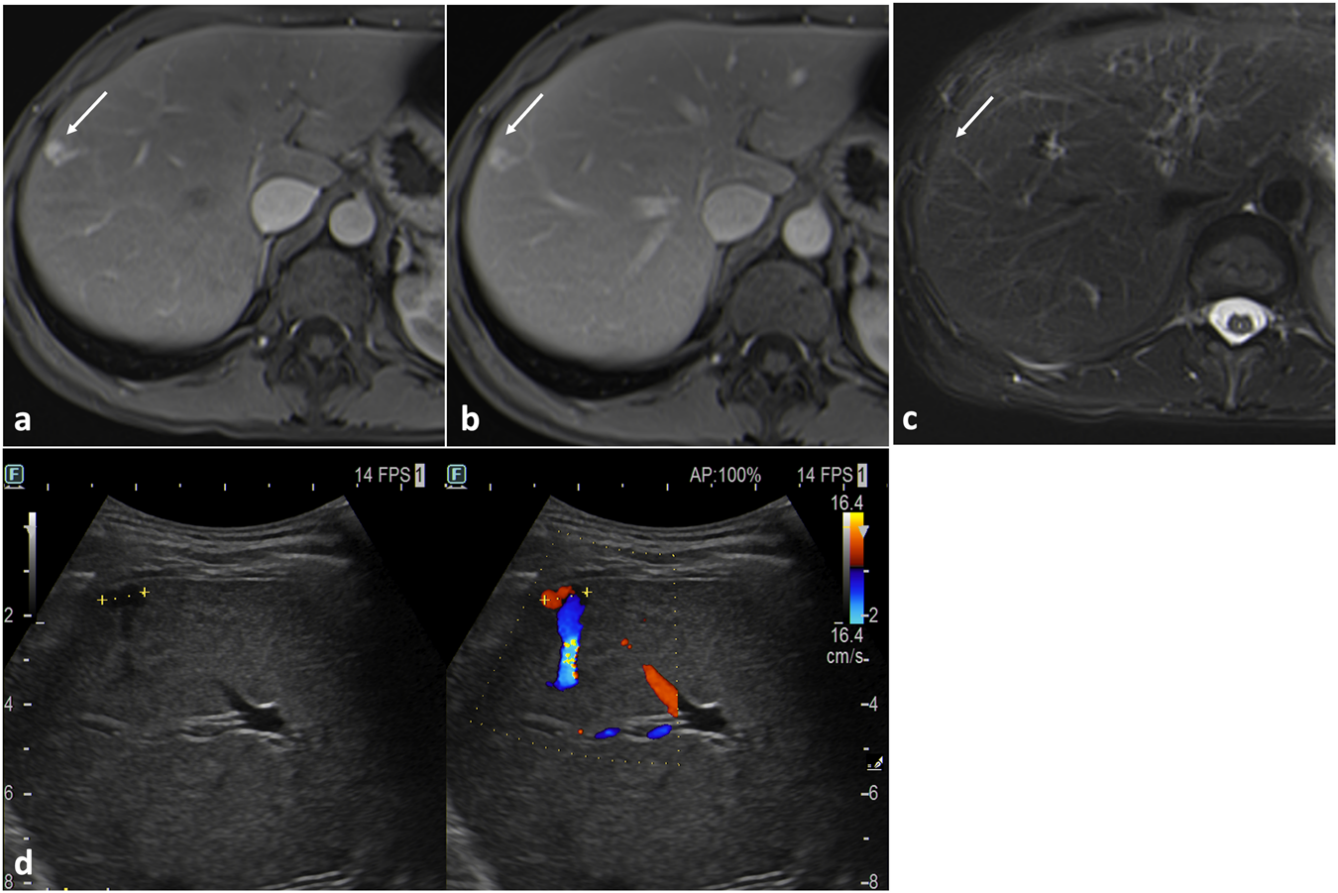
Artero-venous fistula. A focal hypervascular nodule (arrow) is appreciable on arterial phase T1-weighted images (a) between an arterial and a portal branch. The nodule shows the same signal intensity of adjacent vessels in portal venous phase (b), but no focal lesion can be delimited on fat-saturated T2-weighted images (c). On US (d) an anechoic round lesion with arterial flow signal on color-Doppler can be observed
